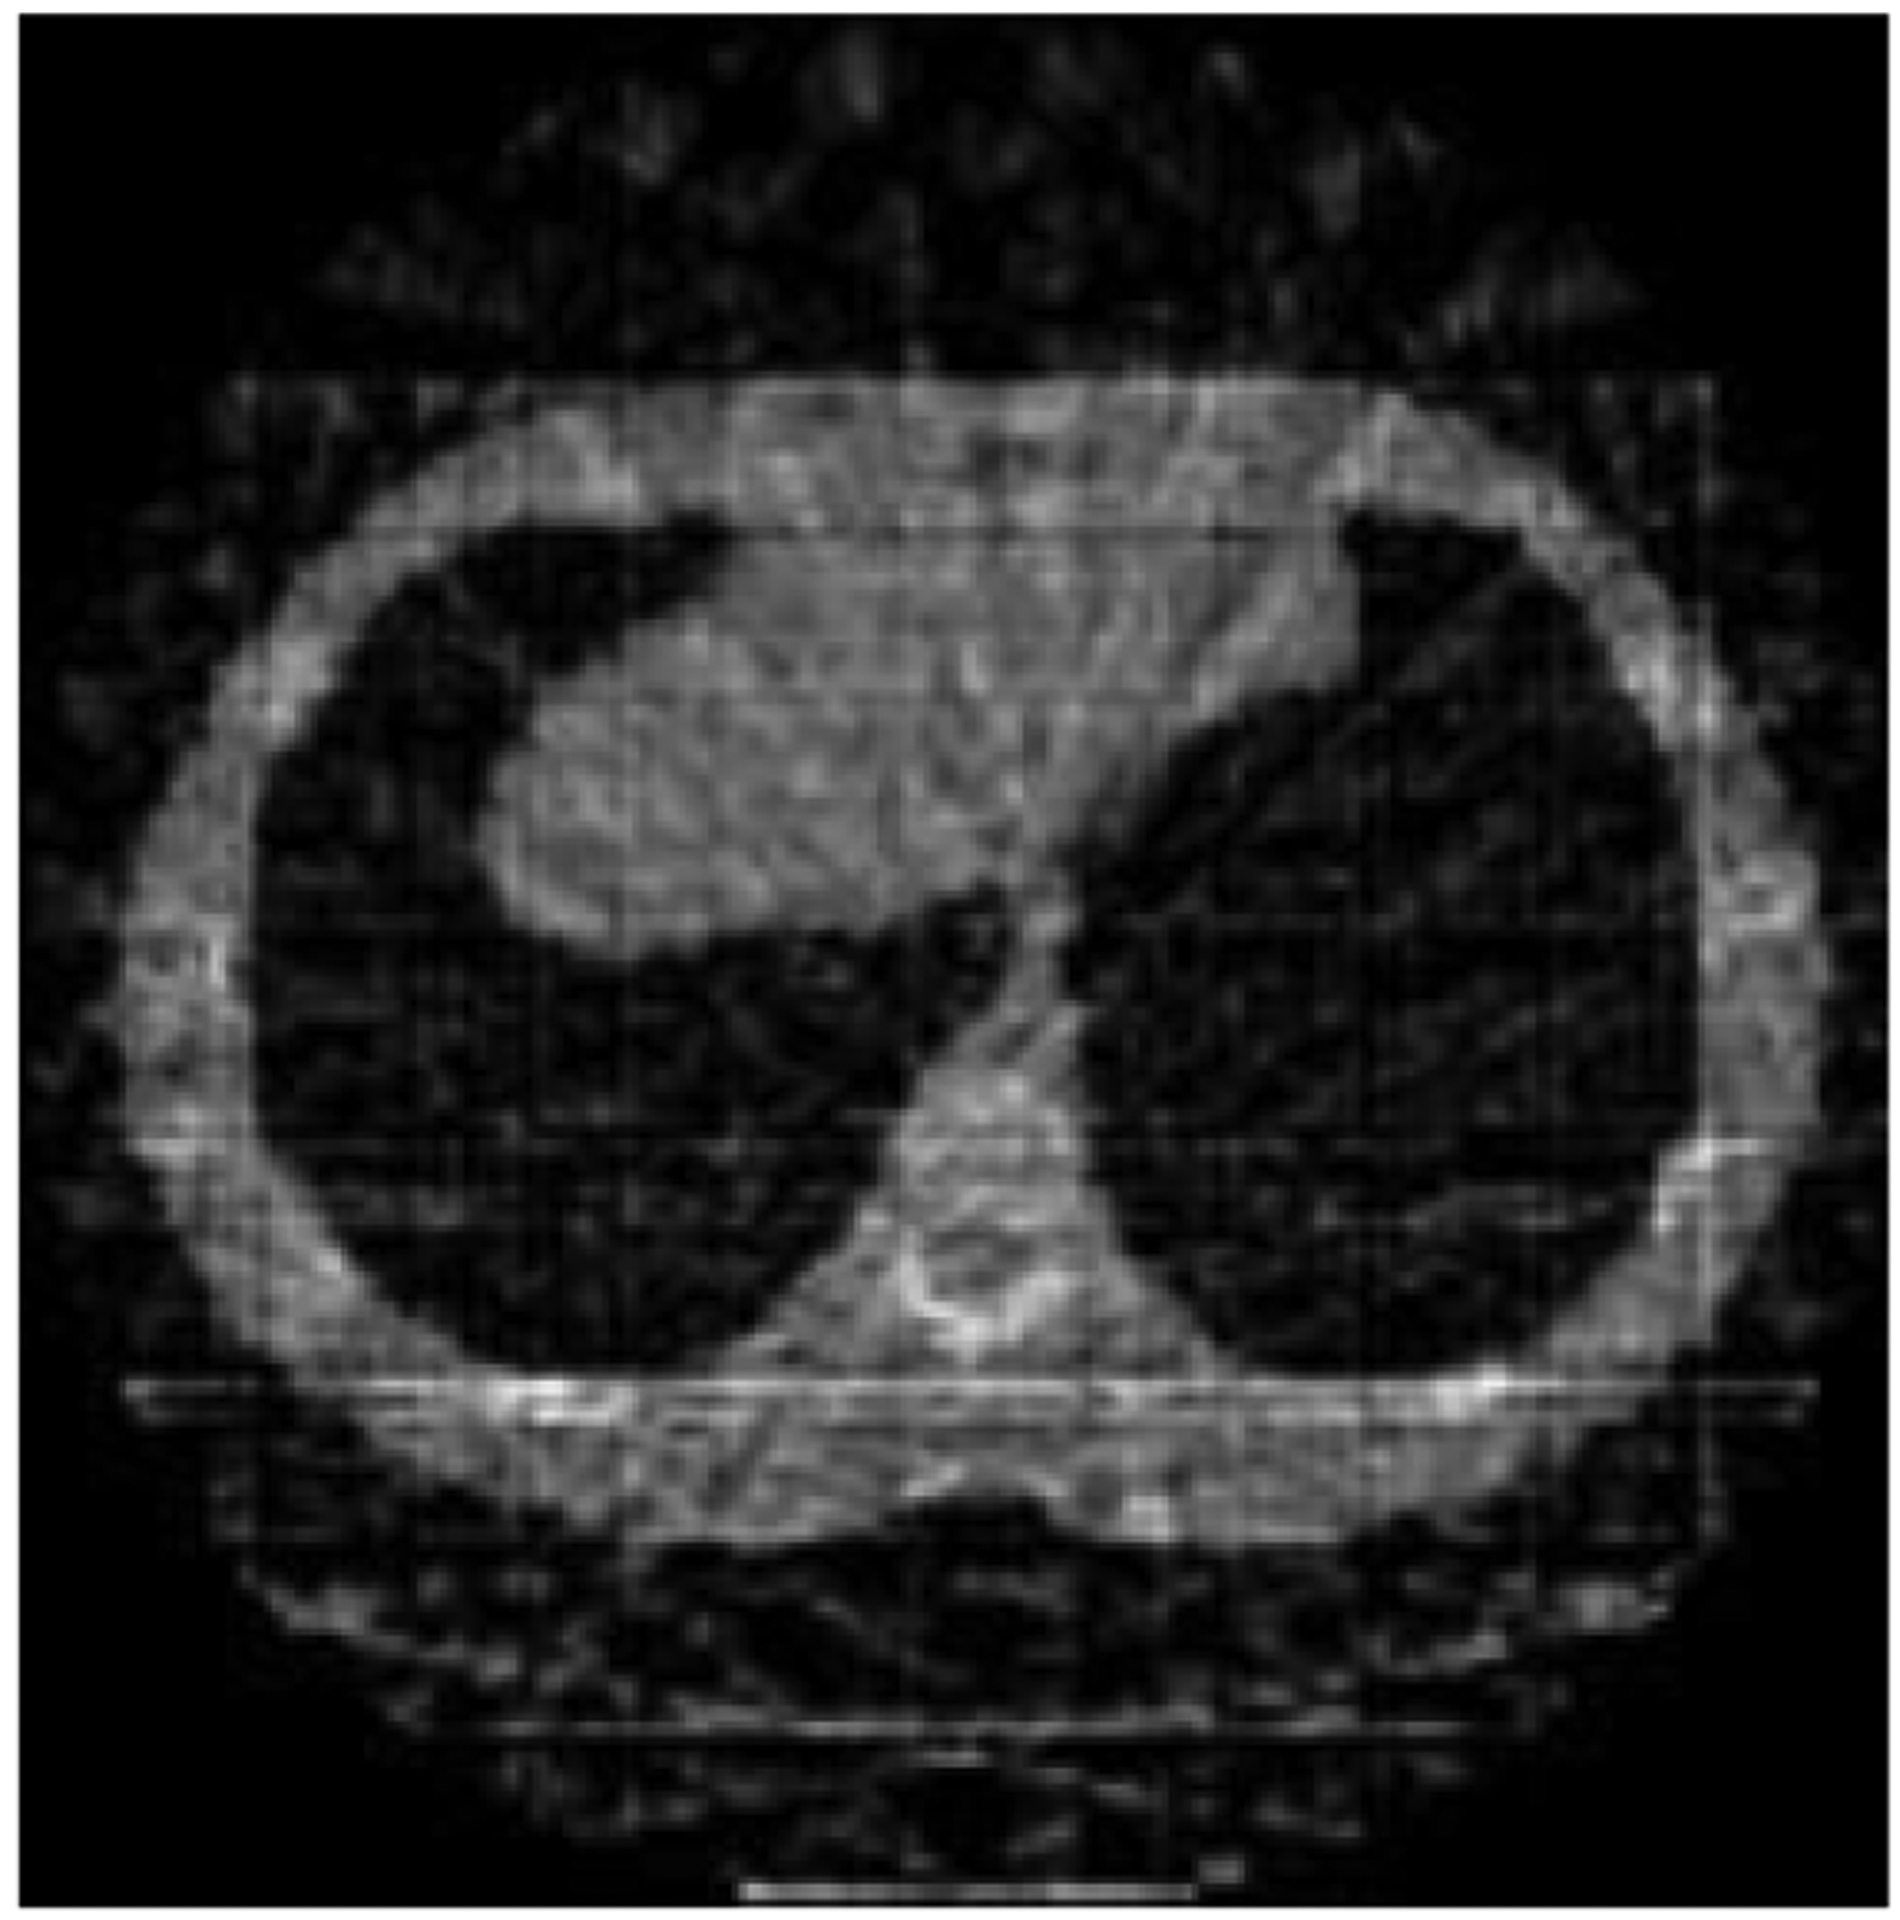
Reconstruction from sparse sonogram.
